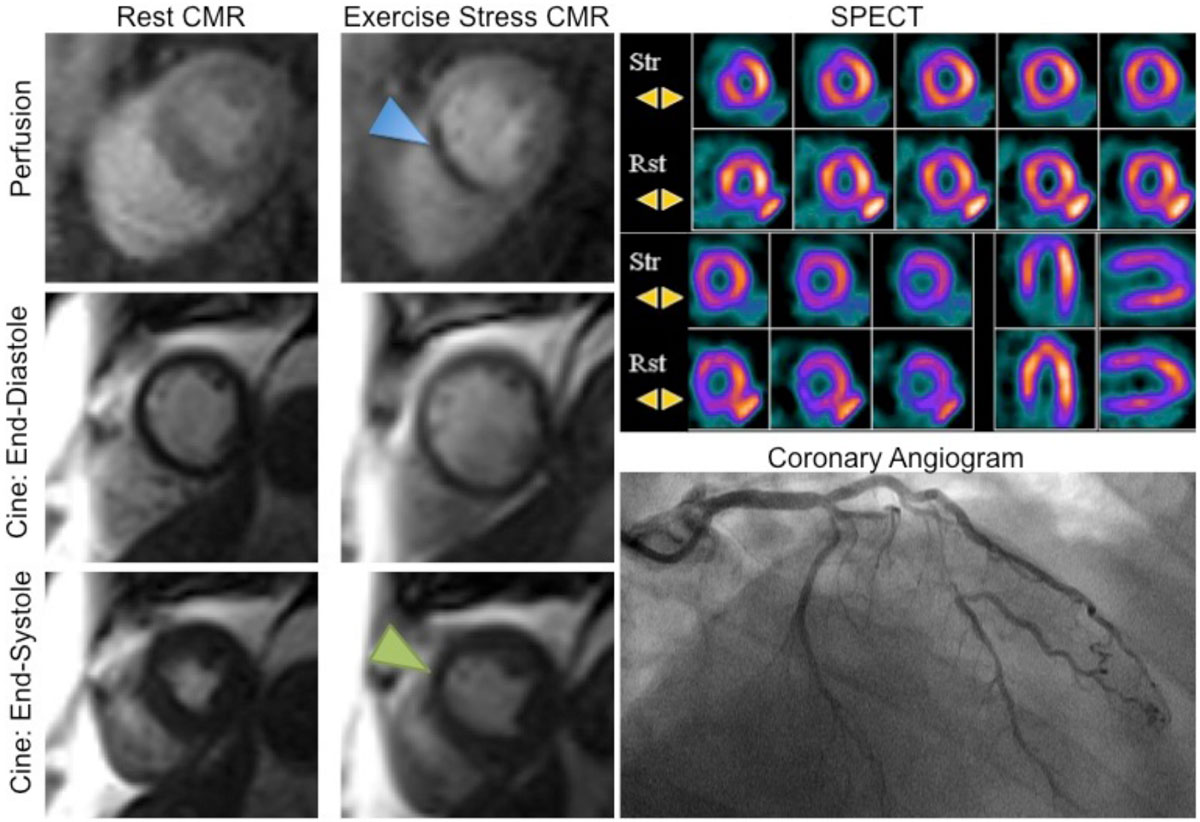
A 59 year-old male referred for exercise SPECT underwent the hybrid treadmill stress CMR-SPECT protocol. While SPECT (upper right color image) suggested a perfusion abnormality, cine and perfusion CMR clearly showed both wall motion and perfusion abnormalities present only on stress images (blue and yellow arrowheads, respectively), with viable myocardium by LGE (not shown). Invasive angiography (lower right) confirmed high-grade left coronary artery disease.
